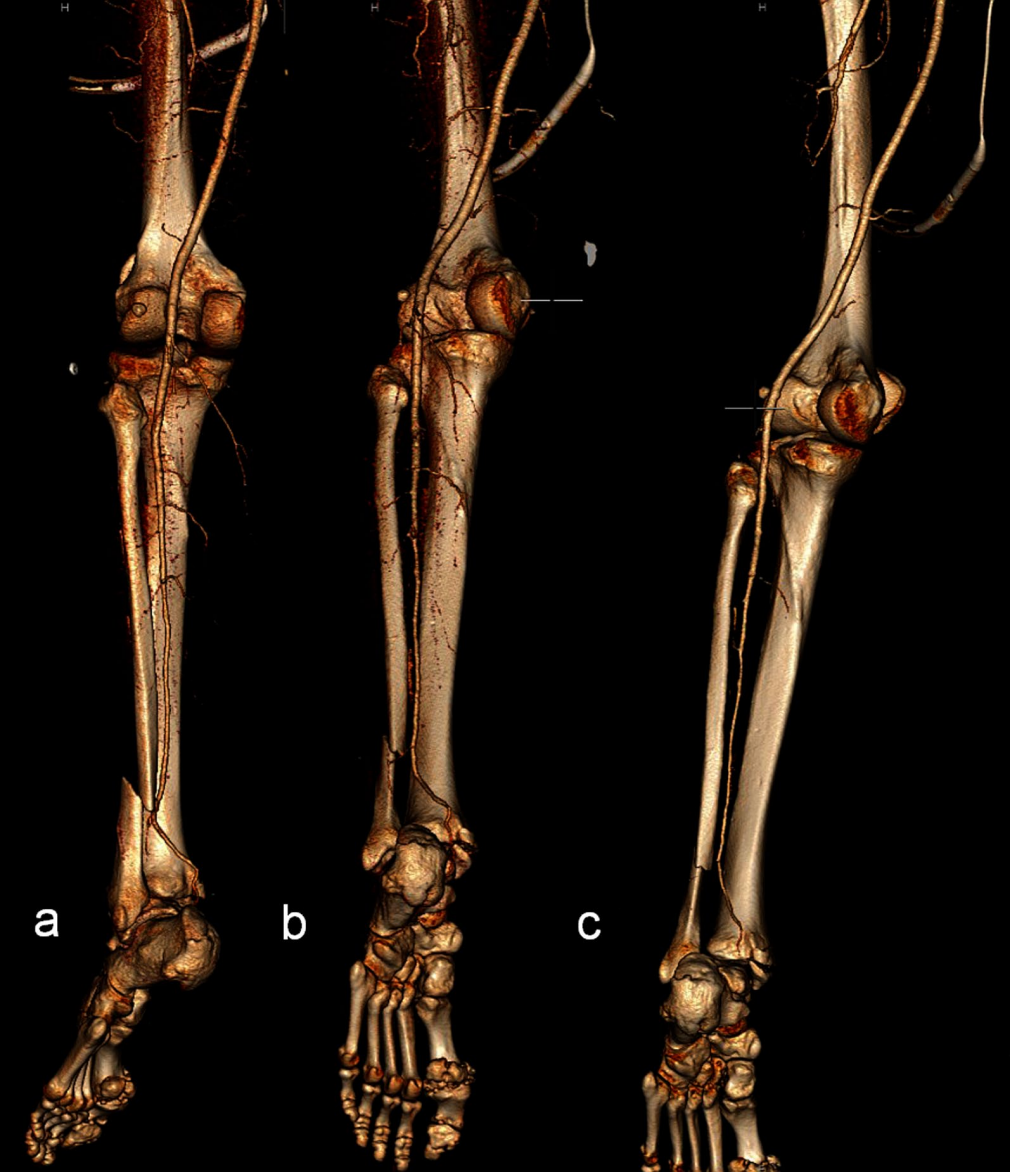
a–c Images from a three-dimensional reconstruction series of left lower limb CT angiogram, demonstrating the absence of both tibial arteries and course of the PAM along the usual course of the peroneal artery proximally, then the PAM courses posterior to the distal tibia and then behind the medial malleolus where the posterior tibial artery is typically located, and a bimalleolar ankle fracture. a Posterior view with internal rotation, b posterior view, c posterior view with external rotation
